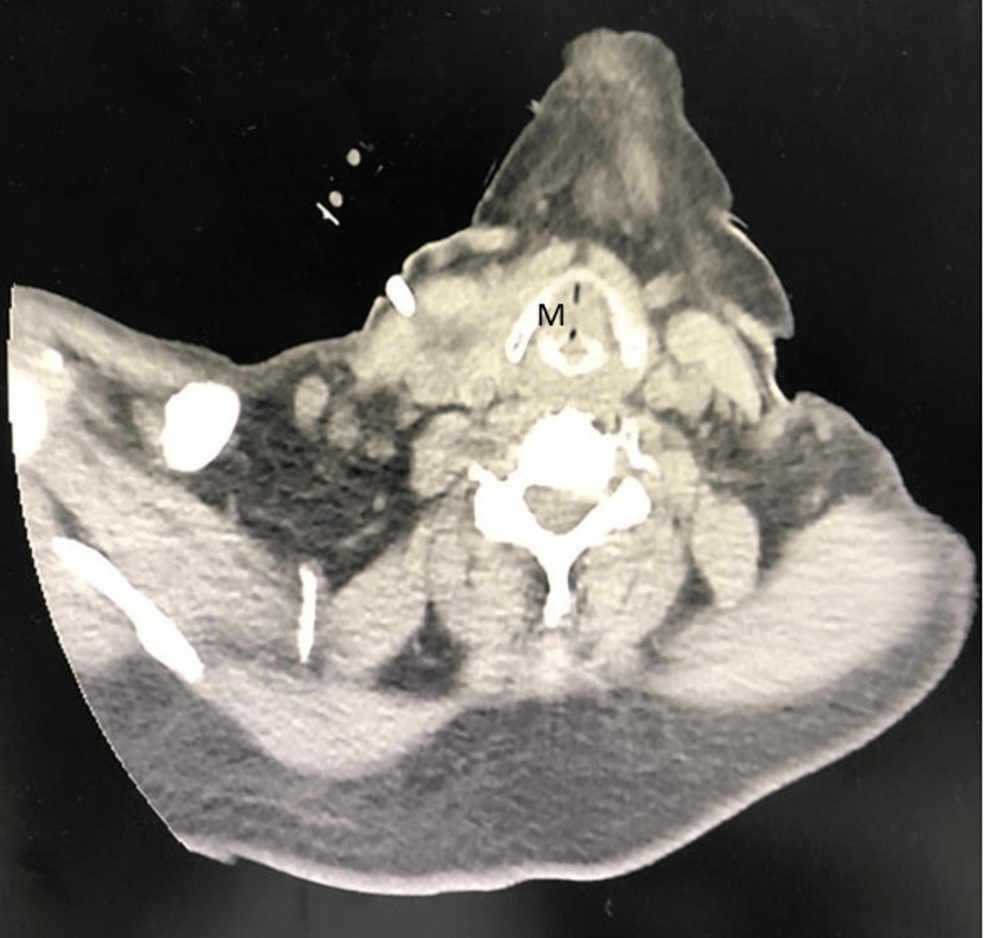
CT of the neck showing a well-defined hypodense submucosal lesion (M) with peripheral enhancement at the posterior subglottic region.
